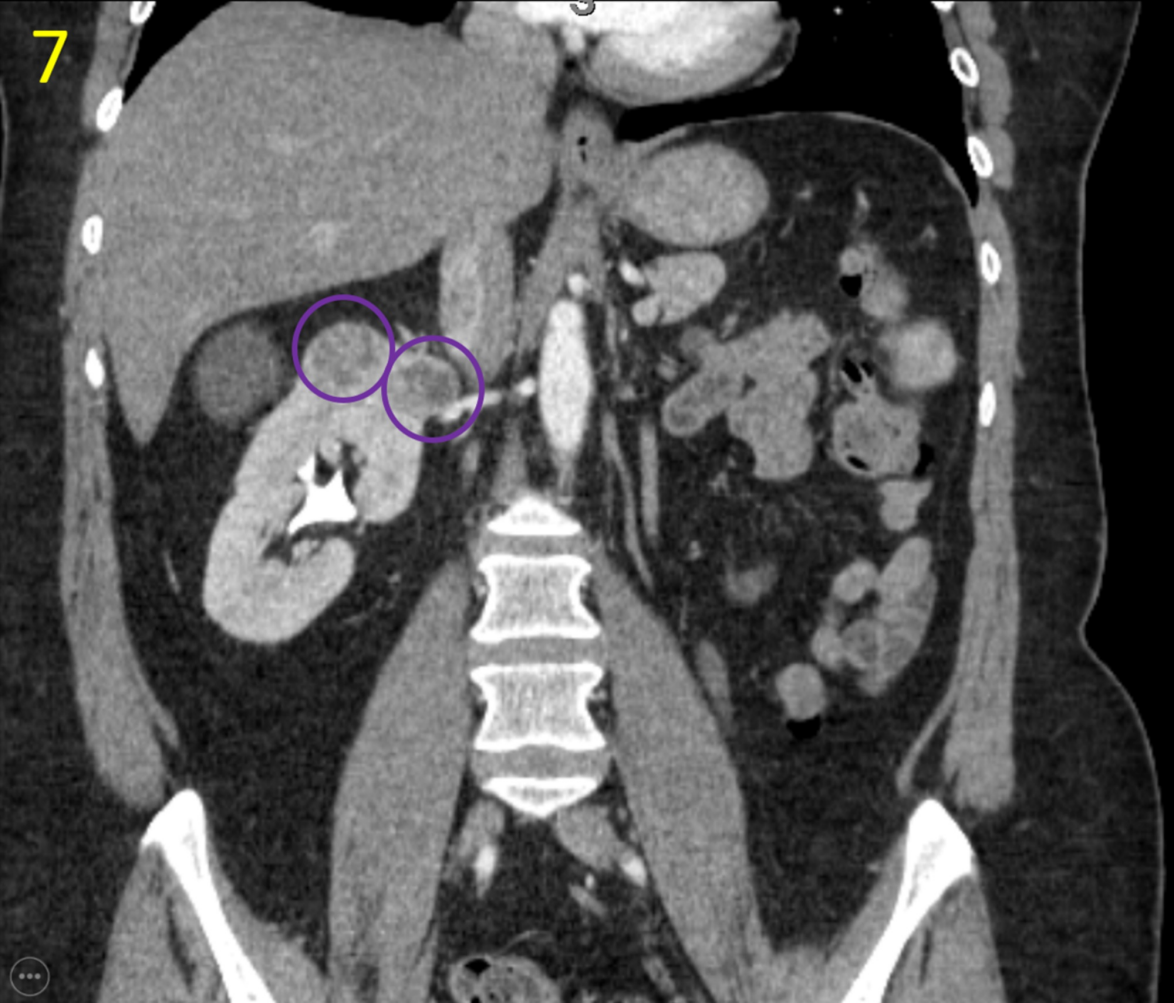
Coronal Abdominal CT This coronal abdominal CT demonstrates several masses in the right kidney suspicious for metastasis (purple circles). There was no invasion of the right renal vein nor any signs of recurrence in the left kidney bed where the radical nephrectomy had been performed.
